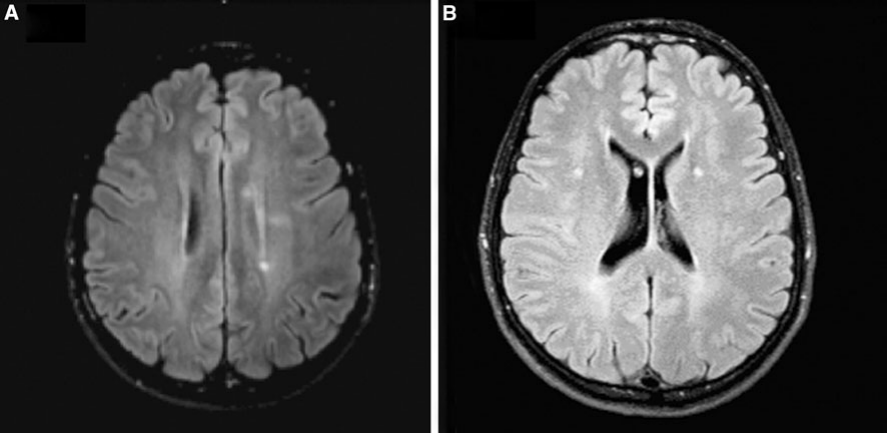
These supratentorial MRI images correspond to the same study as Fig. 2. Both T2-weighted image (a) and FLAIR (b) discovered new periventricular lesions
